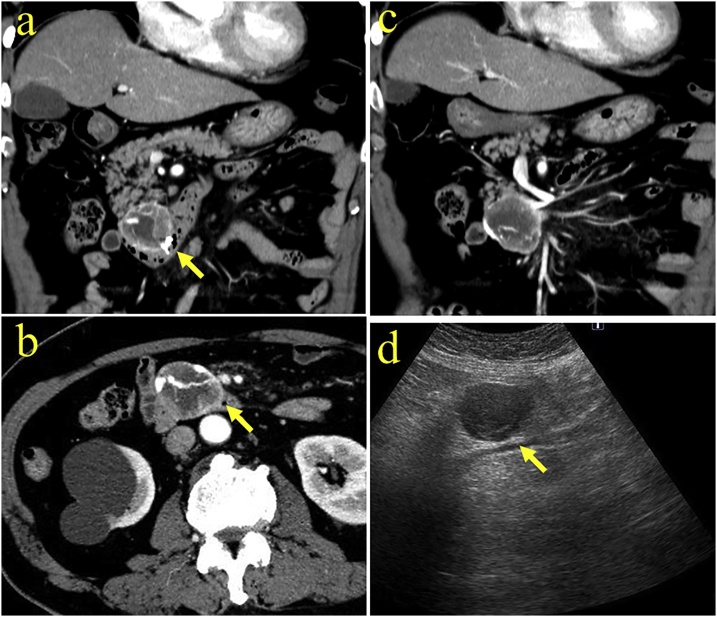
(a) Coronal contrast-enhanced computed tomography (CT) scan showed a heterogeneously enhanced 50 mm tumor in the pancreatic uncus. Arrow indicates clips used for endoscopic hematemesis. (b) Axial contrast enhanced CT scan showed a well-defined exophytic mass (arrow) accompanied by a rich network of feeding vessels passing through the mass. (c) The tumor was fed by the inferior pancreaticoduodenal artery. (d) Abdominal ultrasonography showed a 45 × 30 mm tumor in the head of the pancreas with a smooth surface and simple low-echogenicity, without pancreatic ductal dilatation (arrow).
